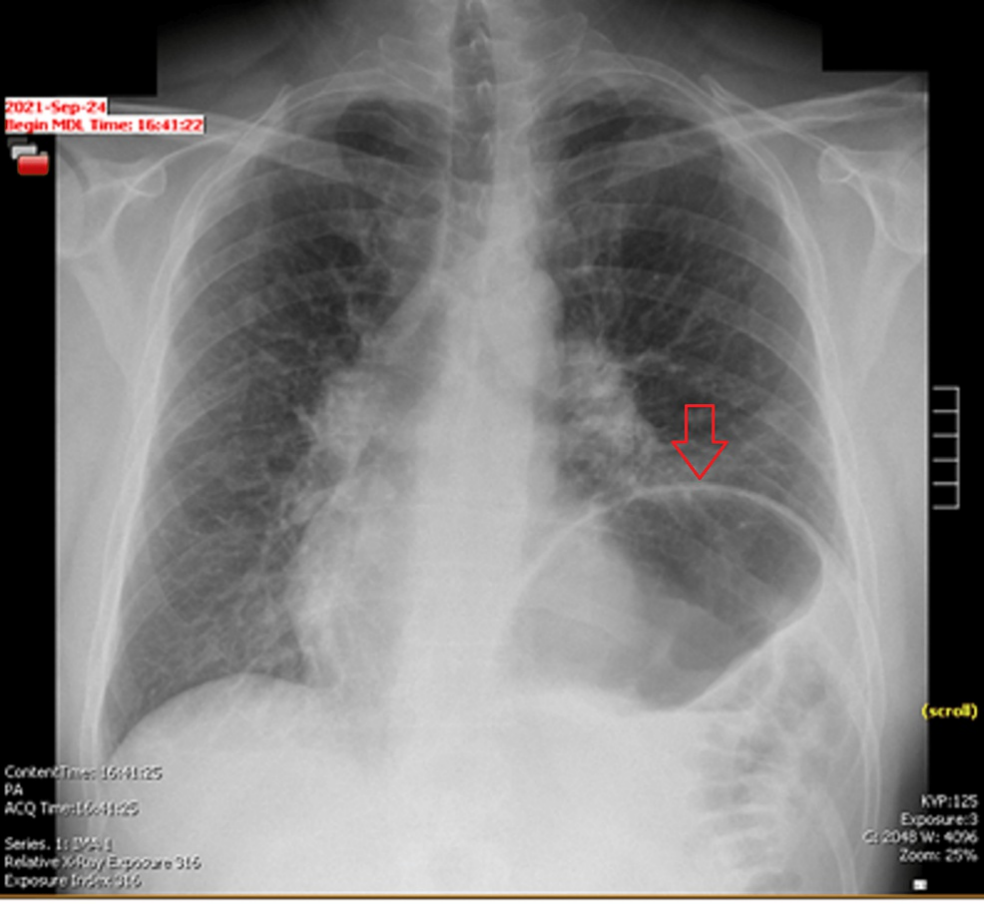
Initial chest X-ray showing left hemidiaphragmatic paralysis
